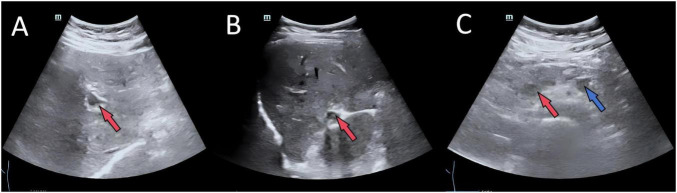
Doppler ultrasound examination confirmed the presence of partial thrombosis within the left portal vein branch (A), the right portal vein branch (B), the superior mesenteric vein [(C), red arrow], and the splenic vein [(C), blue arrow].
